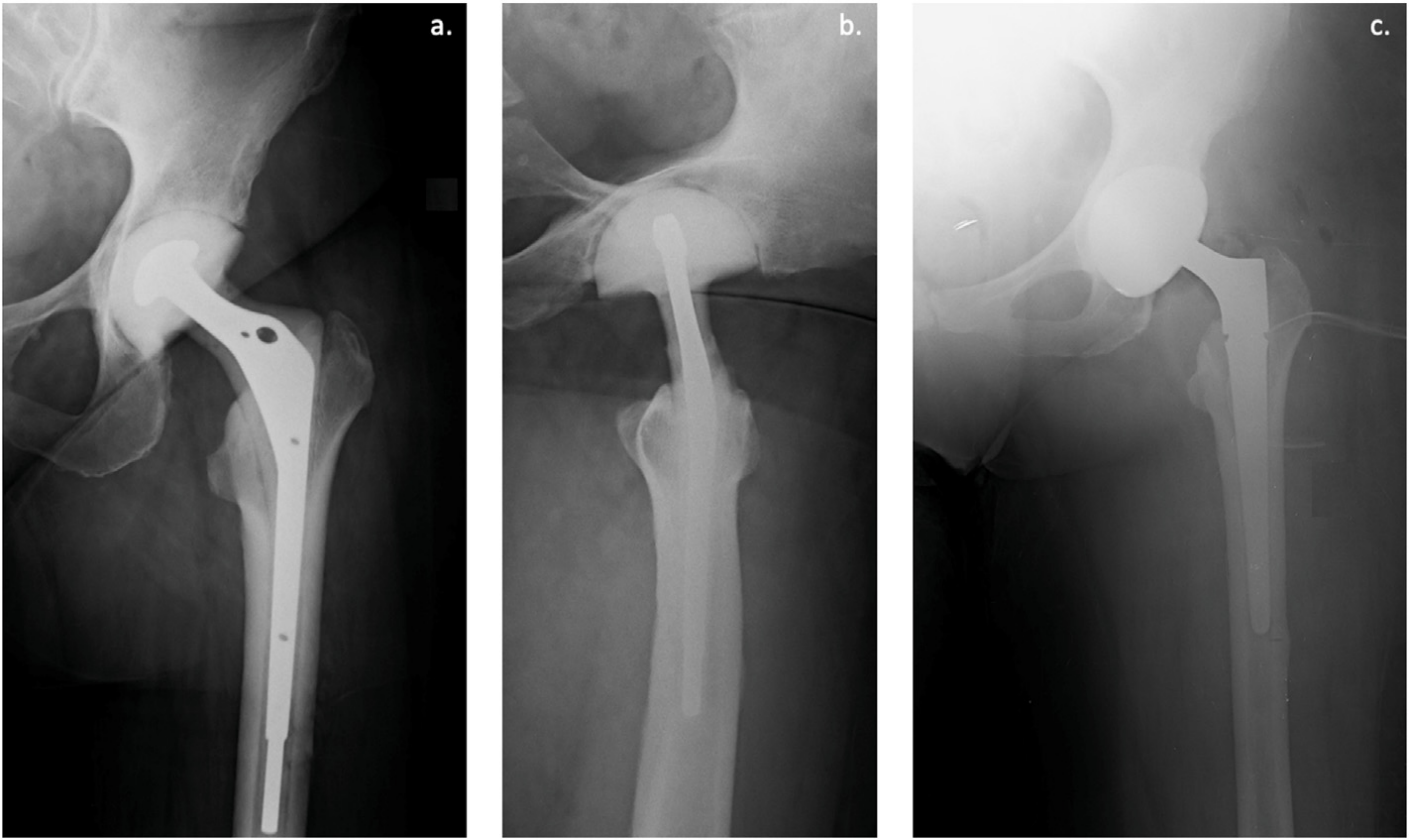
Fig. 4a: An anteroposterior (AP) X-ray of the left hip shows an implanted Spaceflex in the immediate postoperative during the interval period. Fig. 4b: An axial X-ray of the left hip shows an implanted Spaceflex in the immediate postoperative during the interval period. Fig. 4c: An AP X-ray of the left hip shows an implanted Spaceflex in the immediate postoperative reimplantation at the second stage.
